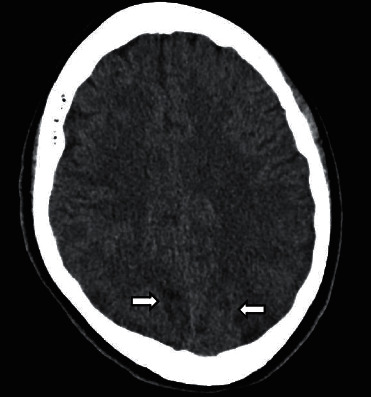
Axial computed tomography image revealing patchy hypodensity in the posterior parietal and occipital lobes, right greater than left. There are few tiny foci of hyperdense hemorrhage seen in areas of edema bilaterally.
